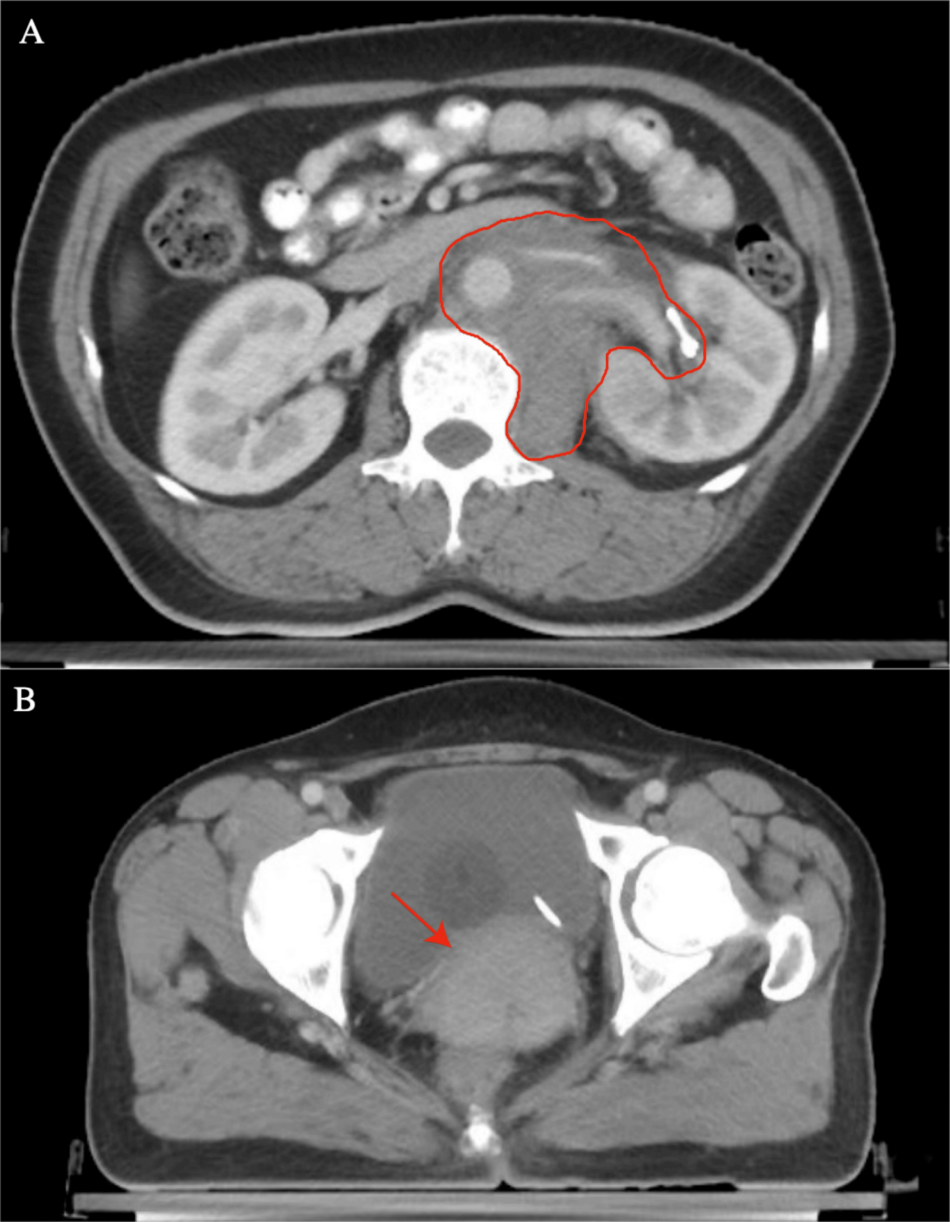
Enhanced computed tomography scan of the patient's abdomen and pelvis at the time of her initial diagnosis with granulocytic sarcoma. (A) An irregular soft tissue mass (outlined by the red line) is observed surrounding the left renal hilum, encasing the left renal hilum structures and the abdominal aorta, with indistinct borders between the mass and the left psoas muscle. (B) A 57×52 mm mass (indicated by the red arrow), with significant localized enhancement on the enhanced scan, is adjacent to the enlarged uterine cervix.
